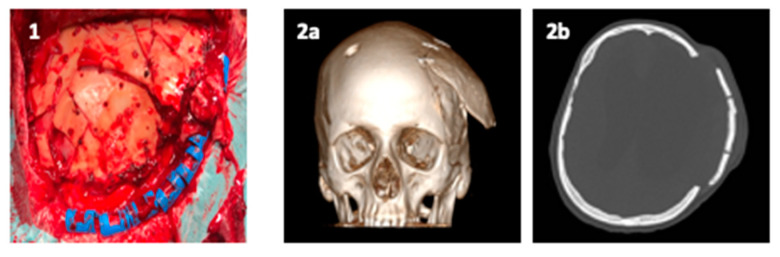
(1) PMMA cranioplasty fractures; (2a) 3D and (2b) axial CT-scan of PMMA cranioplasty displacement.
